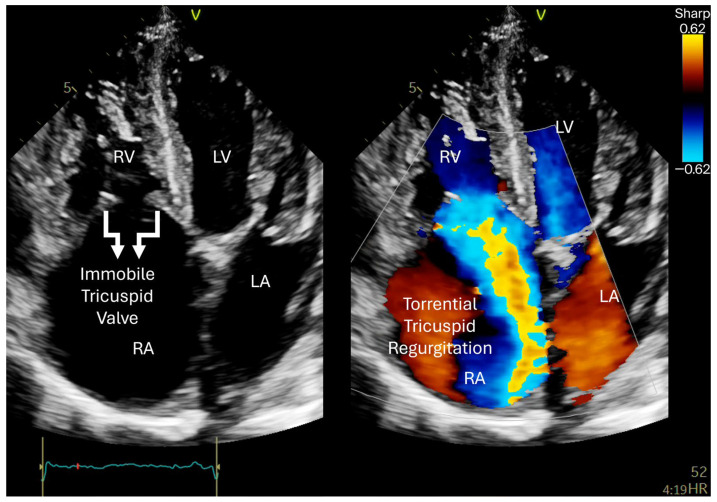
TTE. Apical four-chamber view demonstrating marked right atrium (RA) and right ventricular (RV) dilatation, with thickened, retracted, and immobile tricuspid valve leaflets, resulting in a large coaptation defect. Colour Doppler imaging shows torrential TR, consistent with advanced carcinoid-related tricuspid valve disease. LV: left ventricle; LA: left atrium. See also Video S1.
